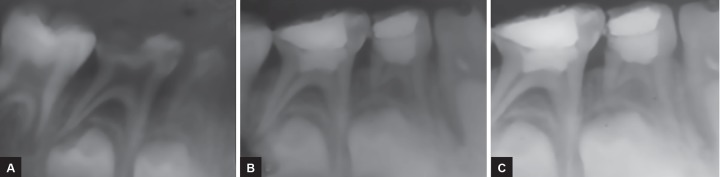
(A) Preoperative radiograph, (B) postoperative radiograph, and (C) 1-year follow-up radiograph
